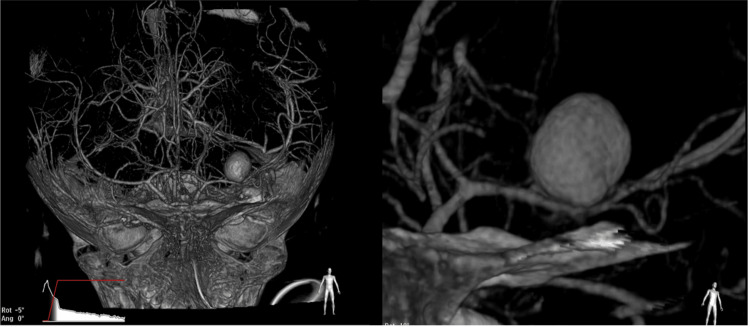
A Vaso-CT scan Right: a dissecting aneurysm/pseudoaneurysm (8 mm × 10 mm × 10 mm) of the left middle cerebral artery, originating from the upper wall of the M1 segment. Left: the same aneurysm amplified.
